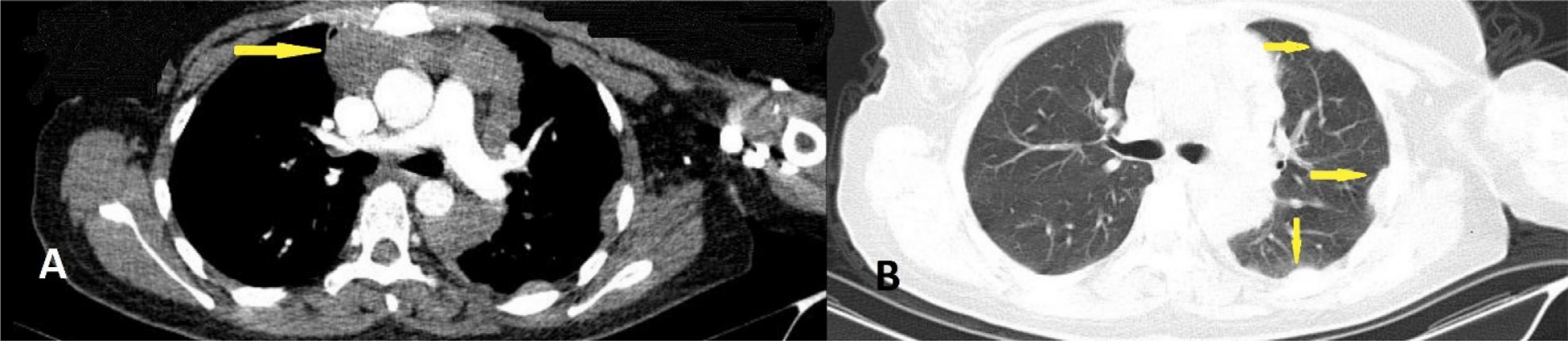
Axial (A, B) chest CT scan with IV contrast. (A): The large lobulated mass in the superior and anterior mediastinal region (yellow arrow). (B): Multiple pleural based lesions in the left hemi thorax, suggestive of pleural metastases (yellow arrows).
